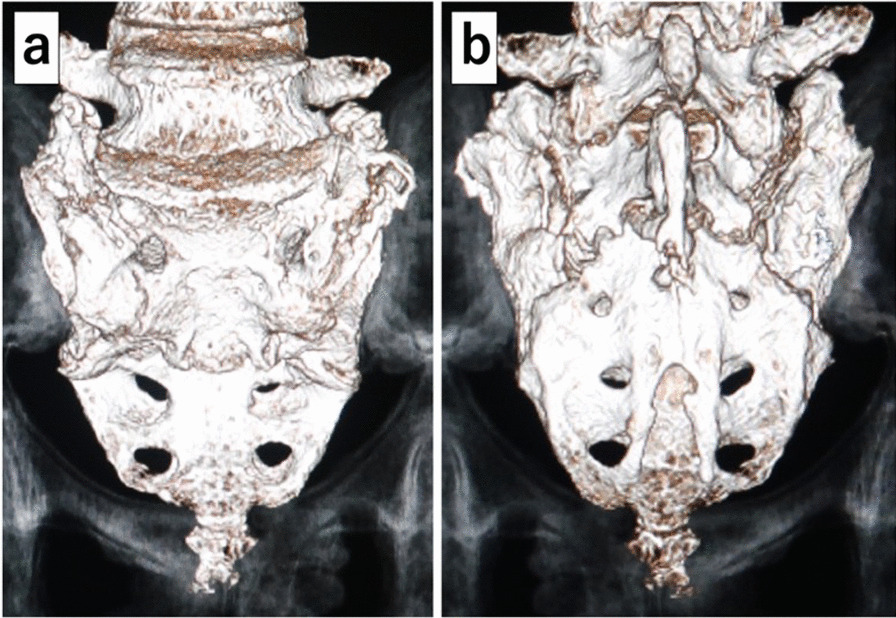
Preoperative three-dimensional computed tomography. a Frontal view, b Posterior view. Both views show an H-shaped sacral fracture (Rommens classification: type IVb)
